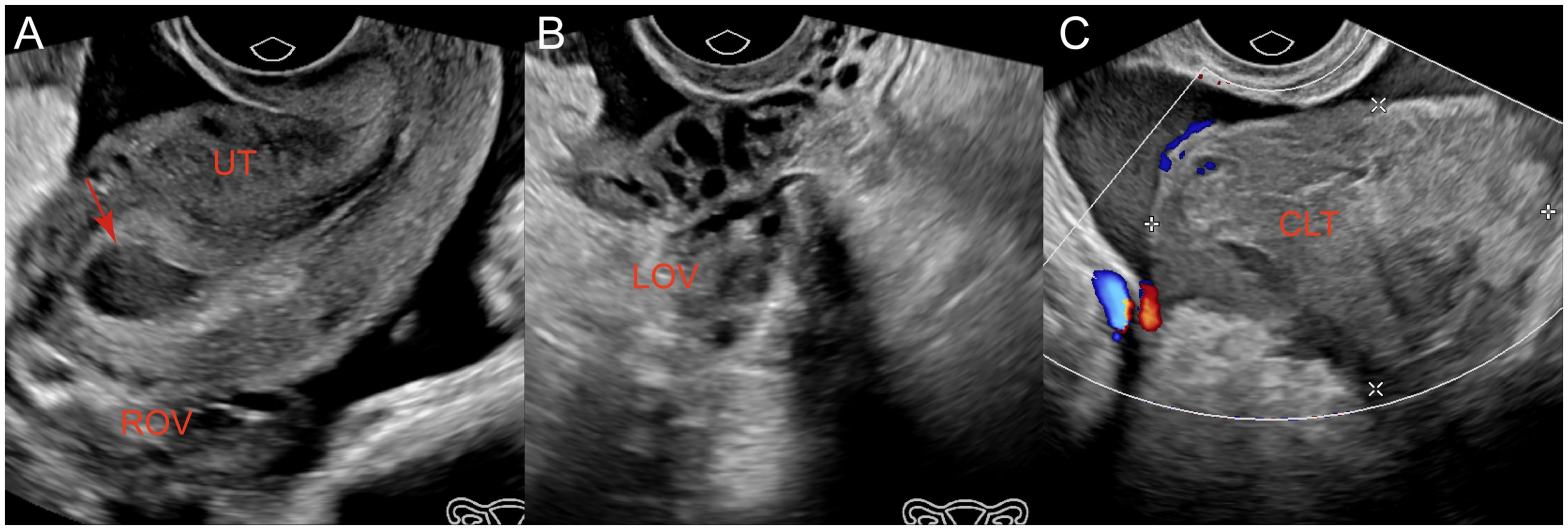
Ultrasonography revealed the following: (A) Uterine effusion (red arrow) and pelvic effusion and no echo of gestational sac within the uterine cavity; (B) No abnormalities detected in the left ovary; (C) A hyperechoic blood clot measuring approximately 66 mm × 47 mm within the pelvic cavity and surrounding effusion. UT, uterus; ROV, right ovary; LOV, left ovary; CLT, blood clot.
